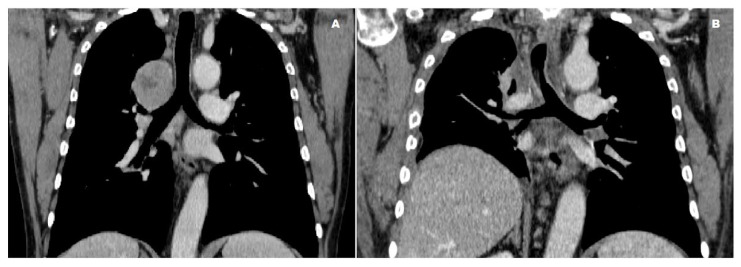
Preoperative (A) and postoperative (B) CT scans of a patient undergoing right carinal upper lobectomy for carcinoid tumor. (A) The preoperative scan reveals a tumor at the tracheobronchial angle without extension to the bronchus intermedius. (B) The postoperative scan shows the reconstructed bronchial tree with restored anatomy.
